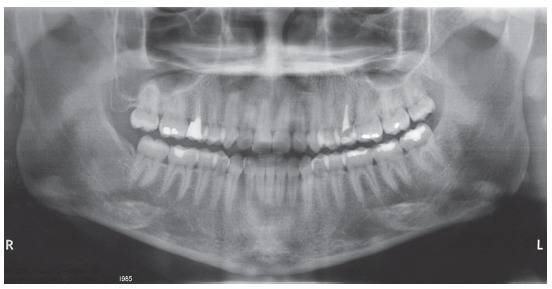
Panoramic radiography two years after treatment completion.
